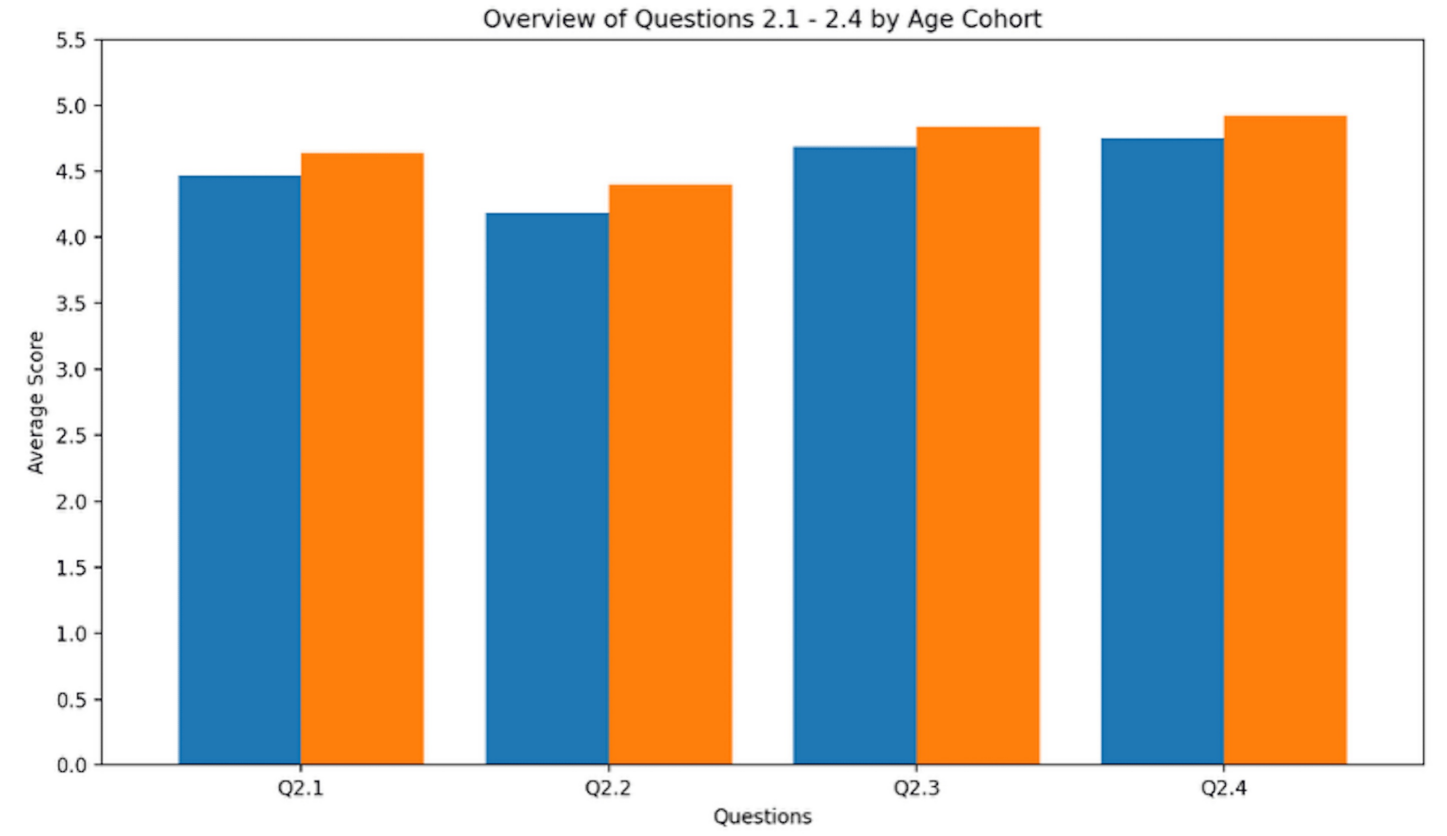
Usability features. Values for usability features are given as mean (SD). Q2.1 - Ultrasound probe usability in virtual environment: junior = 4.47 (0.62); senior = 4.64 (0.57). Q2.2 - Button and control arrangement intuitiveness: junior = 4.19 (0.91); senior = 4.40 (0.76). Q2.3 - Probe positioning capability in virtual environment: junior = 4.69 (0.48); senior = 4.84 (0.37). Q2.4 - Probe handling and operation comfort: junior = 4.75 (0.45); senior = 4.92 (0.28).
